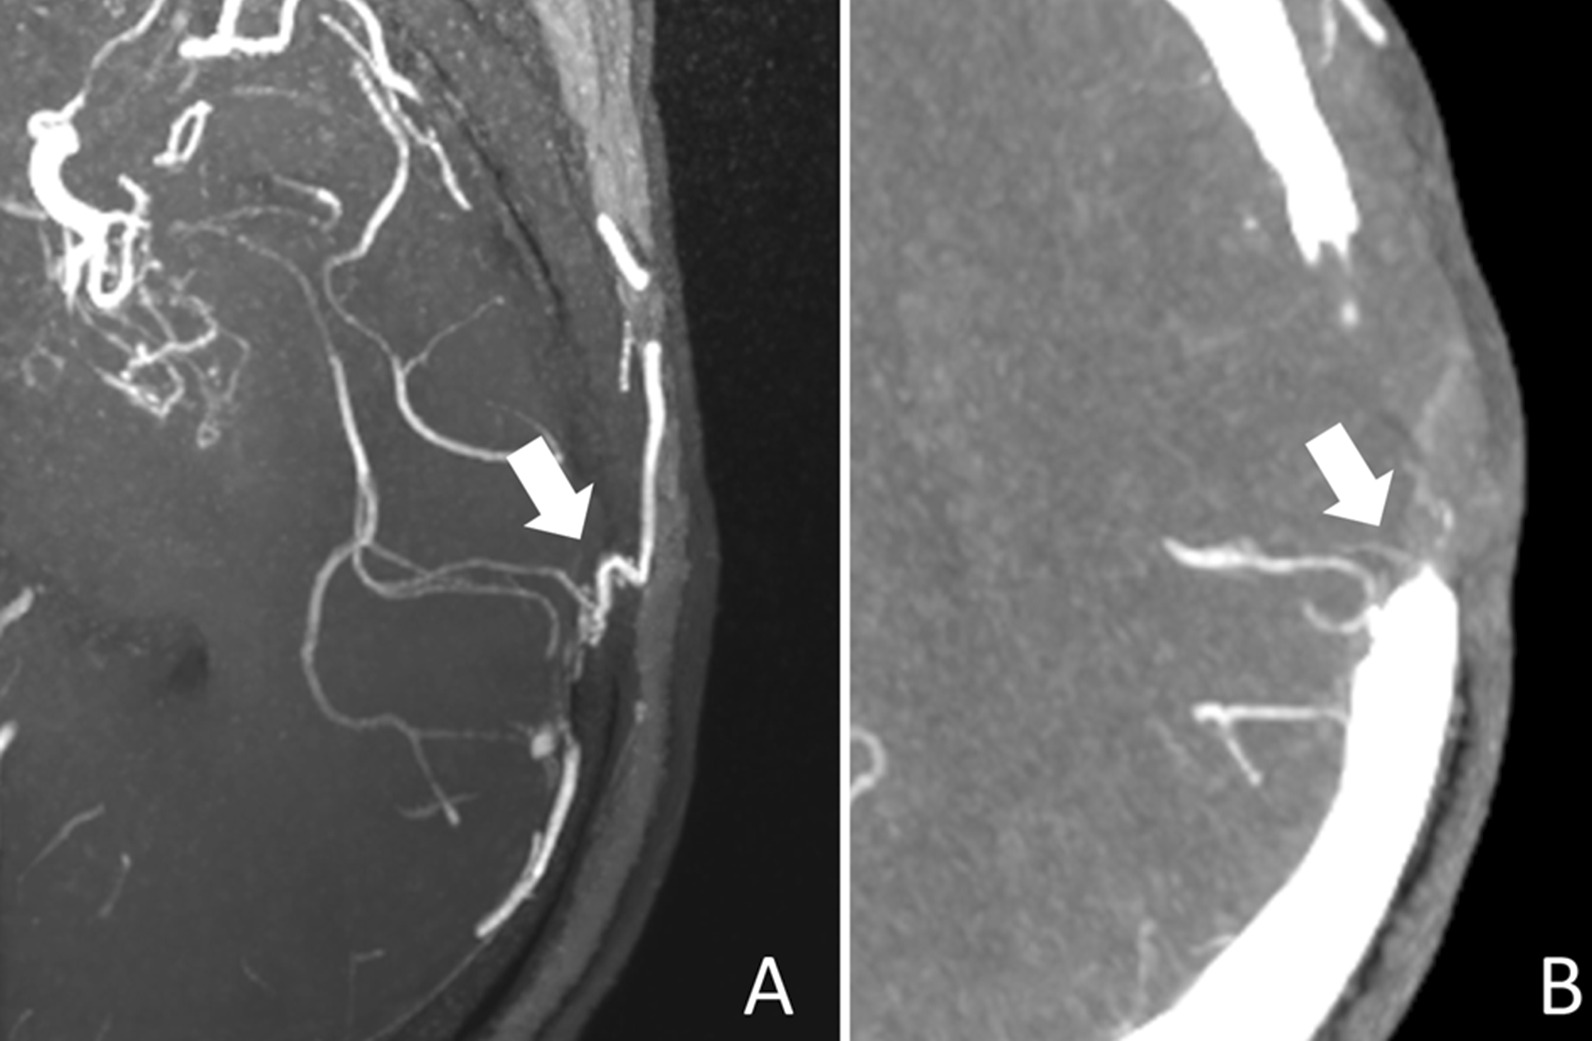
MIP images of a 50-year-old man 17 months after surgical revascularization. The CS TOF-MRA shows the patent anastomosis (A), which is considered occluded on CTA (B). Note the increased background noise and the interference from the skull on CTA
